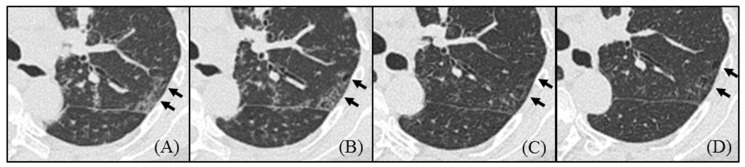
The course of high-resolution computed tomography imaging of case presentation (Case 8). Initial CT showed ground-glass attenuation (A), which regressed after treatment with Tocilizumab (B–D). However, new cysts gradually emerged fromGGA regressed with treatment (B–D).
